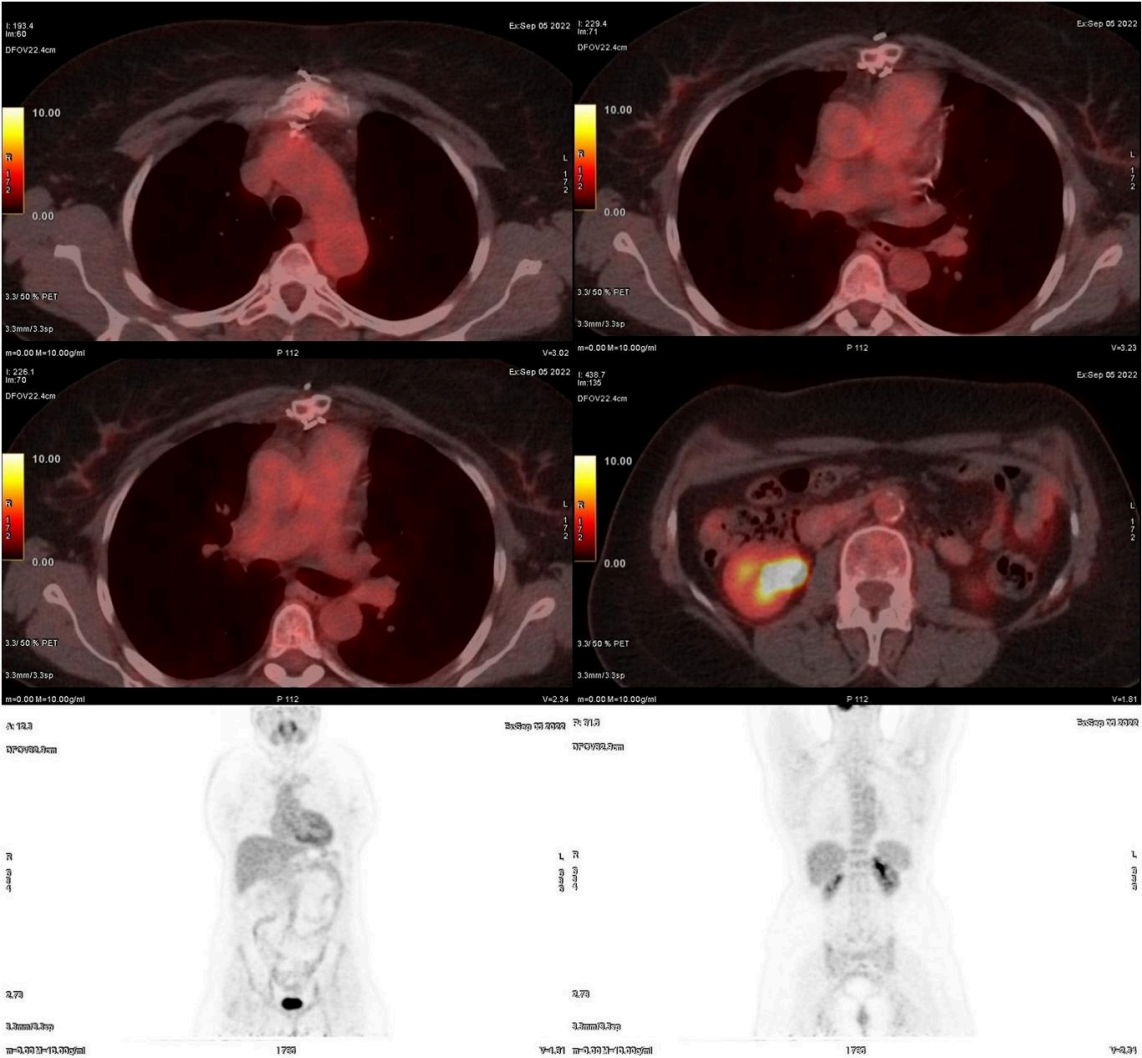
Representative 18F-FDG PET scan showing no evidence of active inflammation. The scan reveals normal physiological uptake of 18F-FDG, with no abnormal areas of increased tracer accumulation typically indicative of inflammatory activity. The absence of hypermetabolic regions suggests that the patient does not have active inflammatory processes at the time of imaging.
